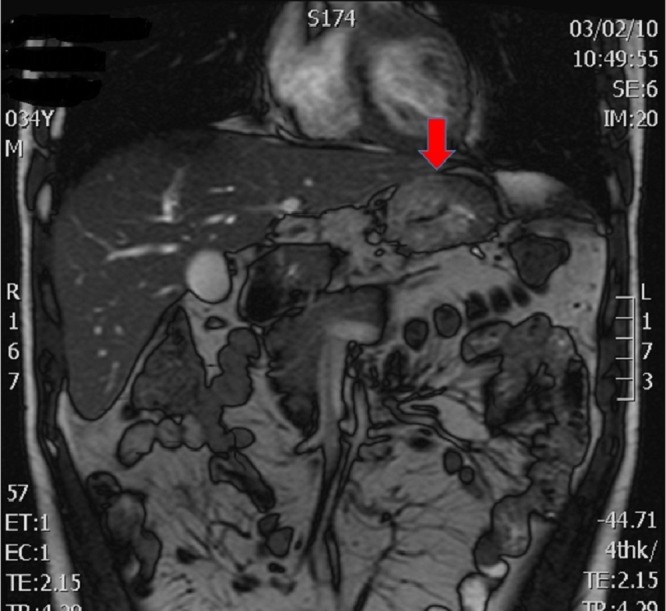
CT and MRI Preoperative Images Coronal MRI - arrow shows primary gastric tumor adjacent to left lobe of liver.
